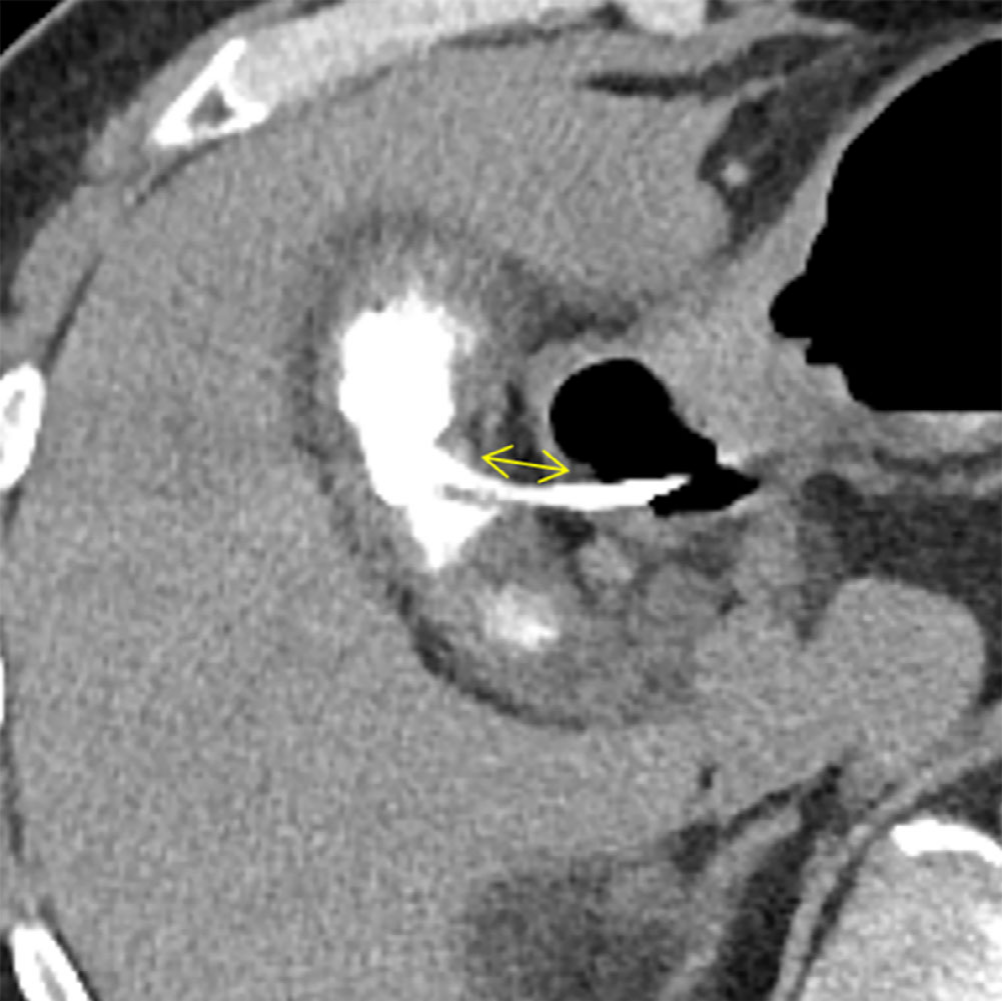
Surveillance for pneumoperitoneum, bile leakage, and length of anastomosis site on post‐procedural CT following EUS‐GBD. Post‐gallbladder lavage was performed twice with 40 mL of contrast‐containing saline. No air or bile leakage is observed around the gallbladder after the procedure. The yellow double‐headed arrow indicates the length of the anastomosis site, measured as the gap between the center of the gallbladder wall and the opposite duodenum. CT, computed tomography; EUS‐GBD, endoscopic ultrasound‐guided gallbladder drainage.
